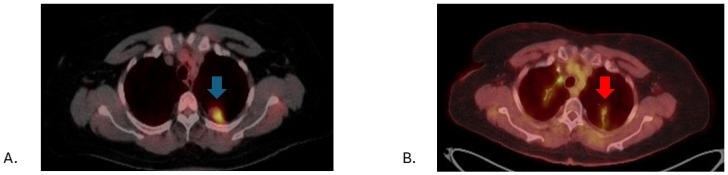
Representative fused PET/CT axial imaging slices from a 68-year-old female with medically inoperable, early-stage squamous cell carcinoma of the left upper lobe lung, pre- and post-lung stereotactic body radiotherapy (SBRT); 34 Gy in 1 fraction on 12/21/2020. (A) Lung cancer with SUVmax of 15.3 (blue arrow) pre-SBRT on 11/18/2010. (B) Lung cancer with SUVmax of 3.6 (red arrow) post-SBRT on 08/14/2017.
